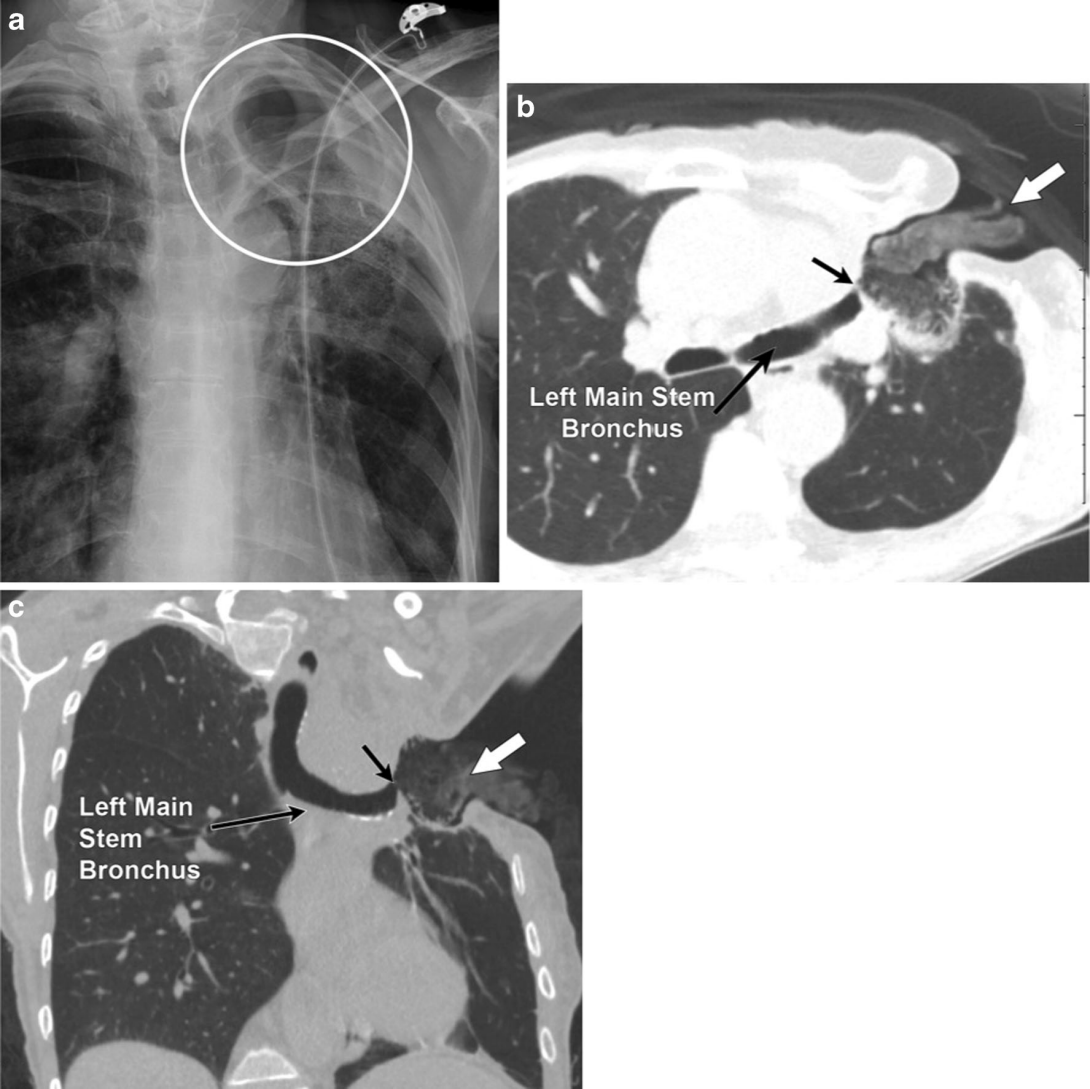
A 67-year-old female; status post Eloesser flap for left empyema. Frontal chest radiograph (a) demonstrates lucency over the left chest apex suggesting pneumothorax (marked by a *circle*). Axial (b) and coronal (c) CT images using lung windows demonstrate the Eloesser flap (*solid white arrow*). In addition, a bronchopleural fistula is also identified (*black arrow*)
